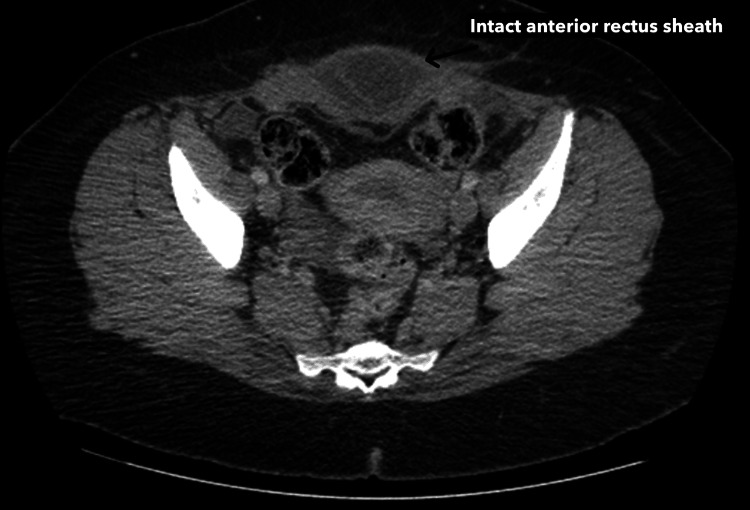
CT scan findings of an incarcerated small bowel loop with intact anterior rectus sheath This axial slide of the CT with oral contrast shows an incarcerated loop of small bowel within the hernia, with an intact anterior rectus sheath evident. The finding of the small bowel between the posterior and anterior rectus sheaths is indicative of an intraparietal hernia.
